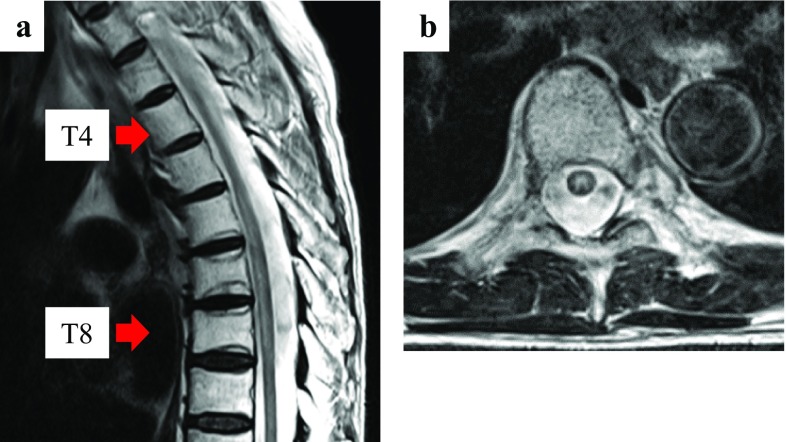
Magnetic resonance imaging 2.5 months after the completion of CRT. a Sagittal: T2 signal hyperintensity lesion between T5 and T8 spinal levels. b Axial: T2 signal hyperintensity lesion in the center of the spinal cord at T7 spinal level
